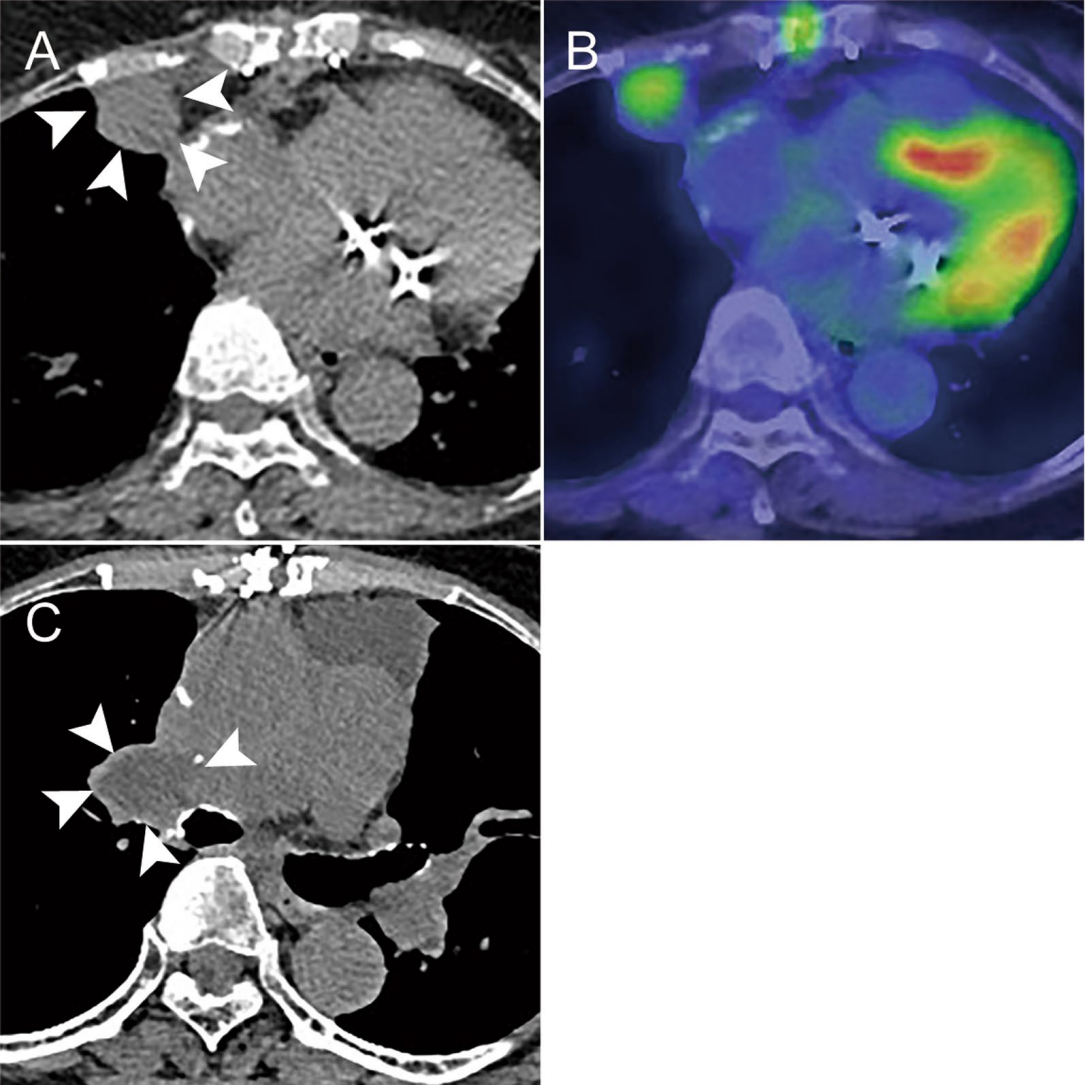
A Computed tomography (CT) obtained three months after the tumor removal showing a nodular lesion adjacent to the chest wall (arrowheads). B The fluorodeoxyglucose (FDG) positron emission tomography CT showing abnormal accumulation of FDG in the lesion. C CT obtained five months after the tumor removal showing a low-density lesion in the right pulmonary artery (arrowheads)
